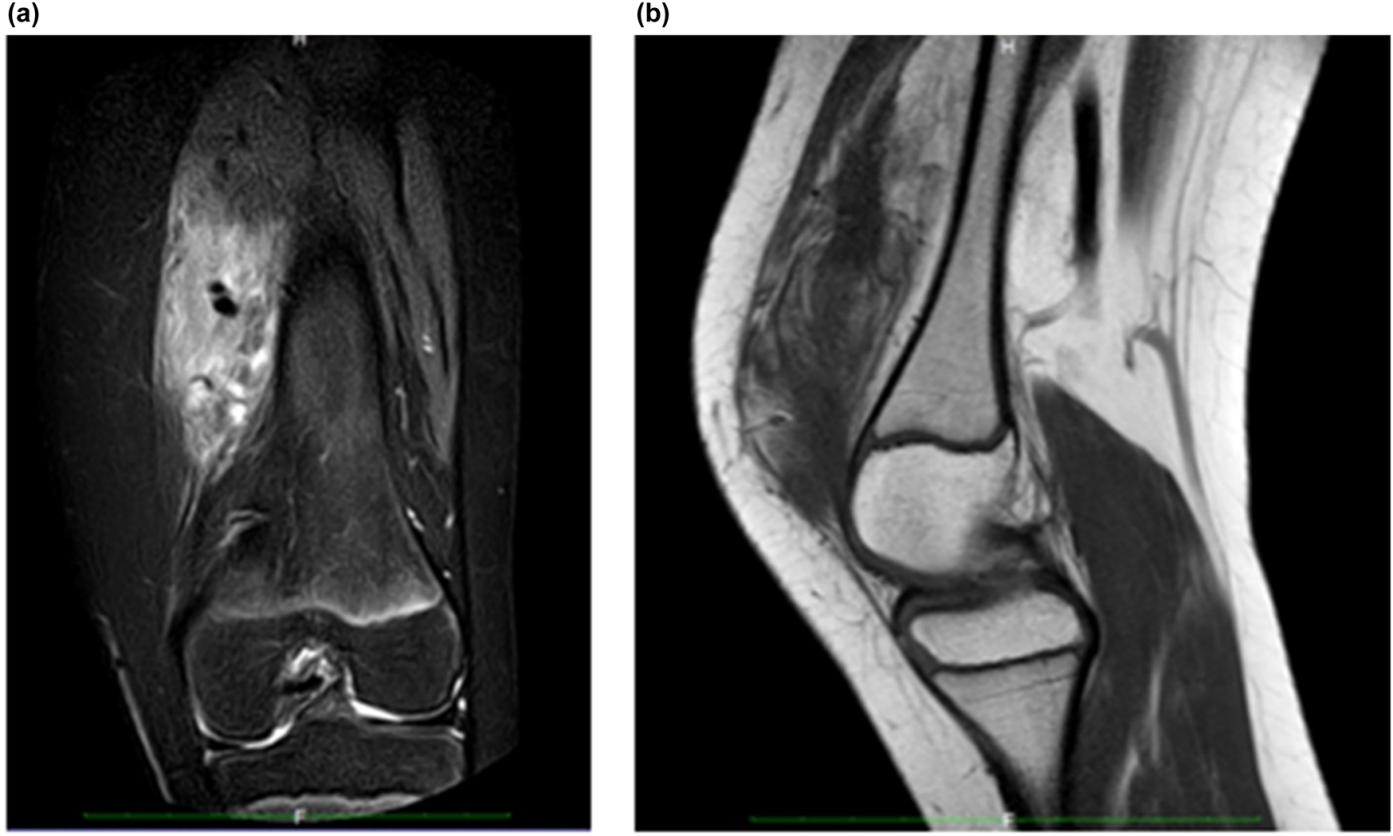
Pre- and postoperative magnetic resonance imaging showing significant improvement in the adipose and vascular components. (a) Preoperative; (b) at 12 months’ postoperative.
